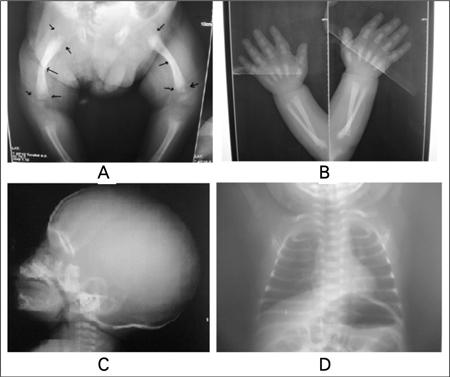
X-rays show bowing and angulation in the long bones,defective mineralization in the epiphyses and metaphyses, demineralizationin the skull, and a normal chest X-ray
